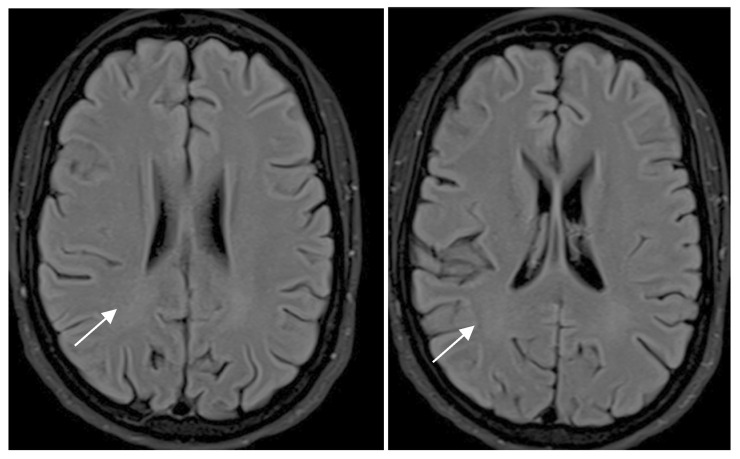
Ax T2-FLAIR MR images demonstrate hazy T2-FLAIR signal hyperintensity (arrows) in the posterior periventricular hyperintensity, presumed to be related to technical differences or technical artifacts. In total, 9 such cases were initially classified as Fazekas 0 and 2, respectively, by each of the readers, with consensus opinion of Fazekas 0.
